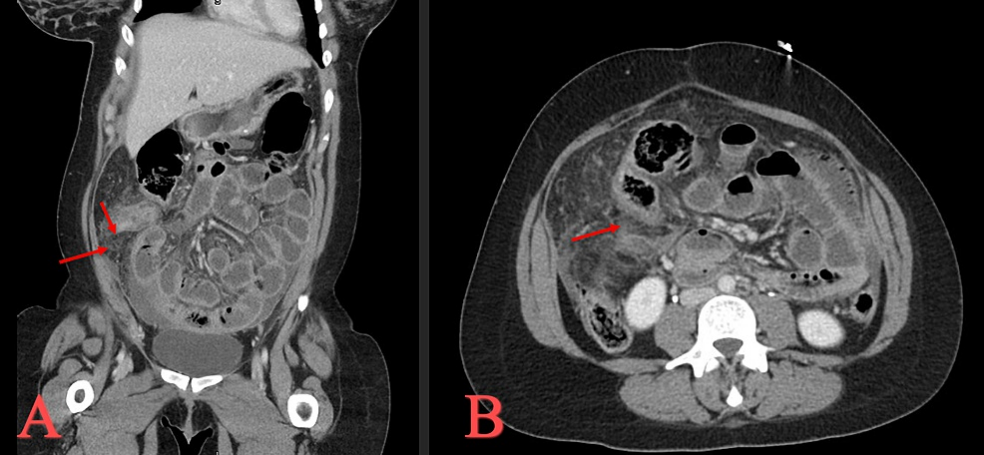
CT of the abdomen and pelvis during the initial presentation. (A) Coronal view with red arrows to demonstrate extensive inflammatory changes found around the appendix. (B) Axial view with red arrow to demonstrate that the appendix is thickened and the walls are not well defined, suggesting ruptured appendicitis.
